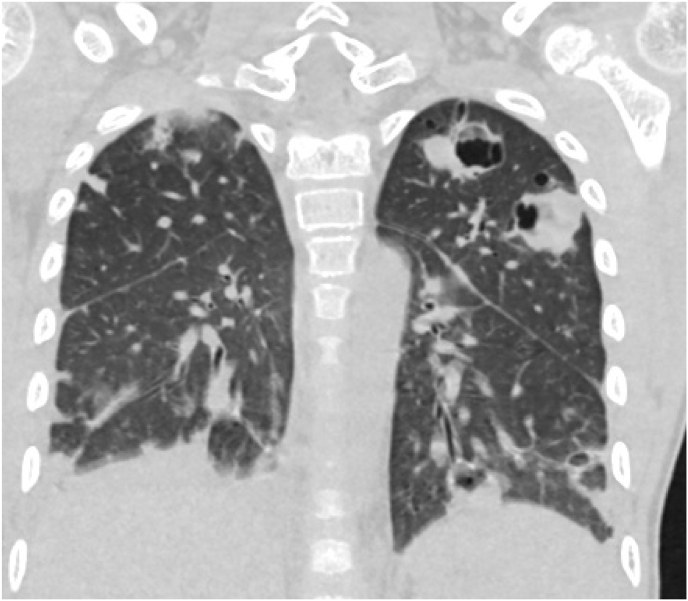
Coronal chest Computed Tomography scan (lung window) showing large irregularly shaped multiple cavities in the left upper lobe with adjacent ground-glass opacity and septal thickening.
